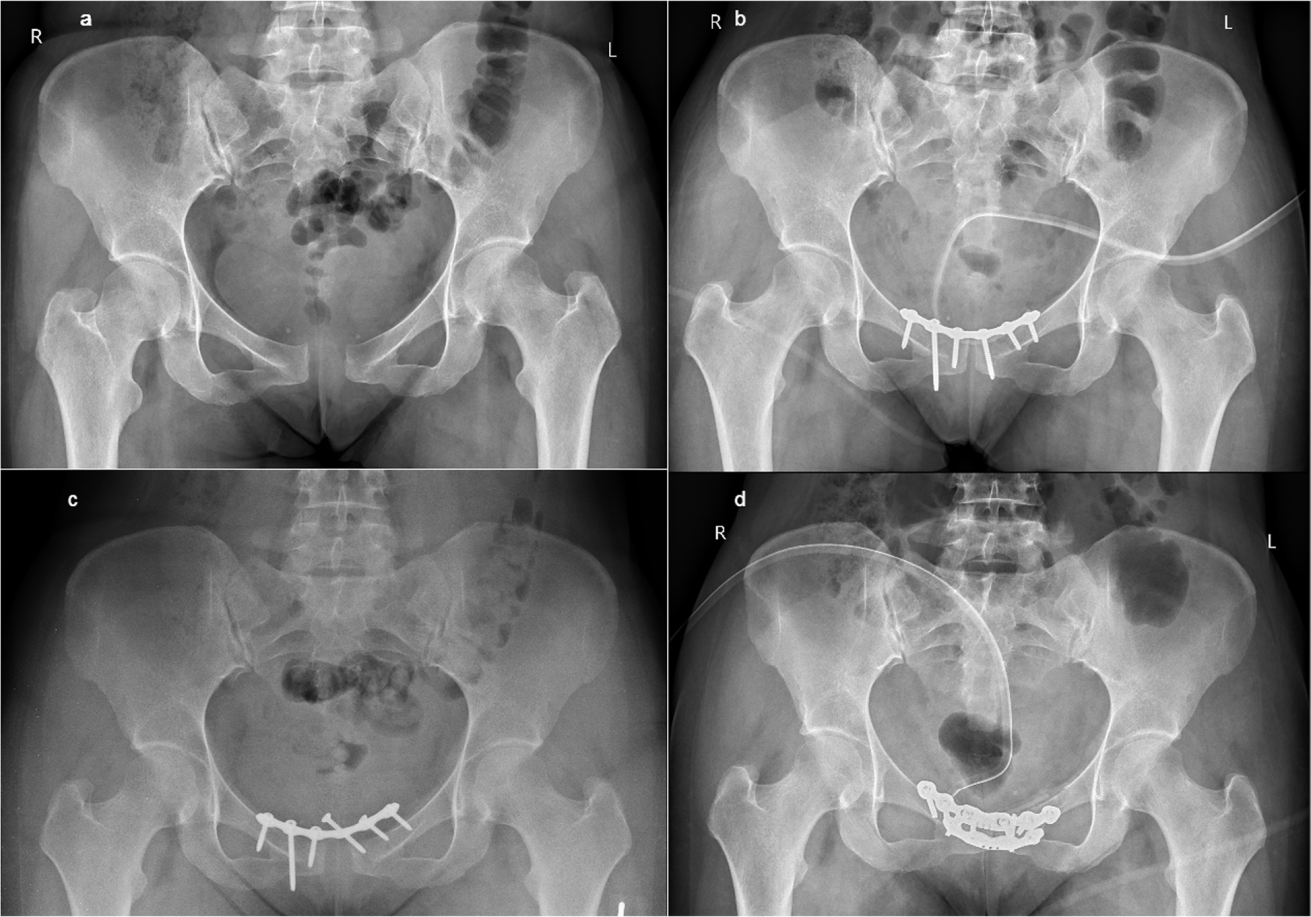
Case 5. A female aged thirty-eight was admitted to the hospital 1 year and 2 months after delivering her child, manifesting with discomfort around the pubic symphysis. a The initial plain radiograph shows a 21 mm wide and 2 mm vertical symphysis diastasis. b Open reduction internal fixation with a dedicated 6-hole plate. c Two months postoperatively, the plain radiograph shows plate failure. d The immediate postoperative film after revision surgery with fixation with two orthogonal 6 and 4-hole plates
